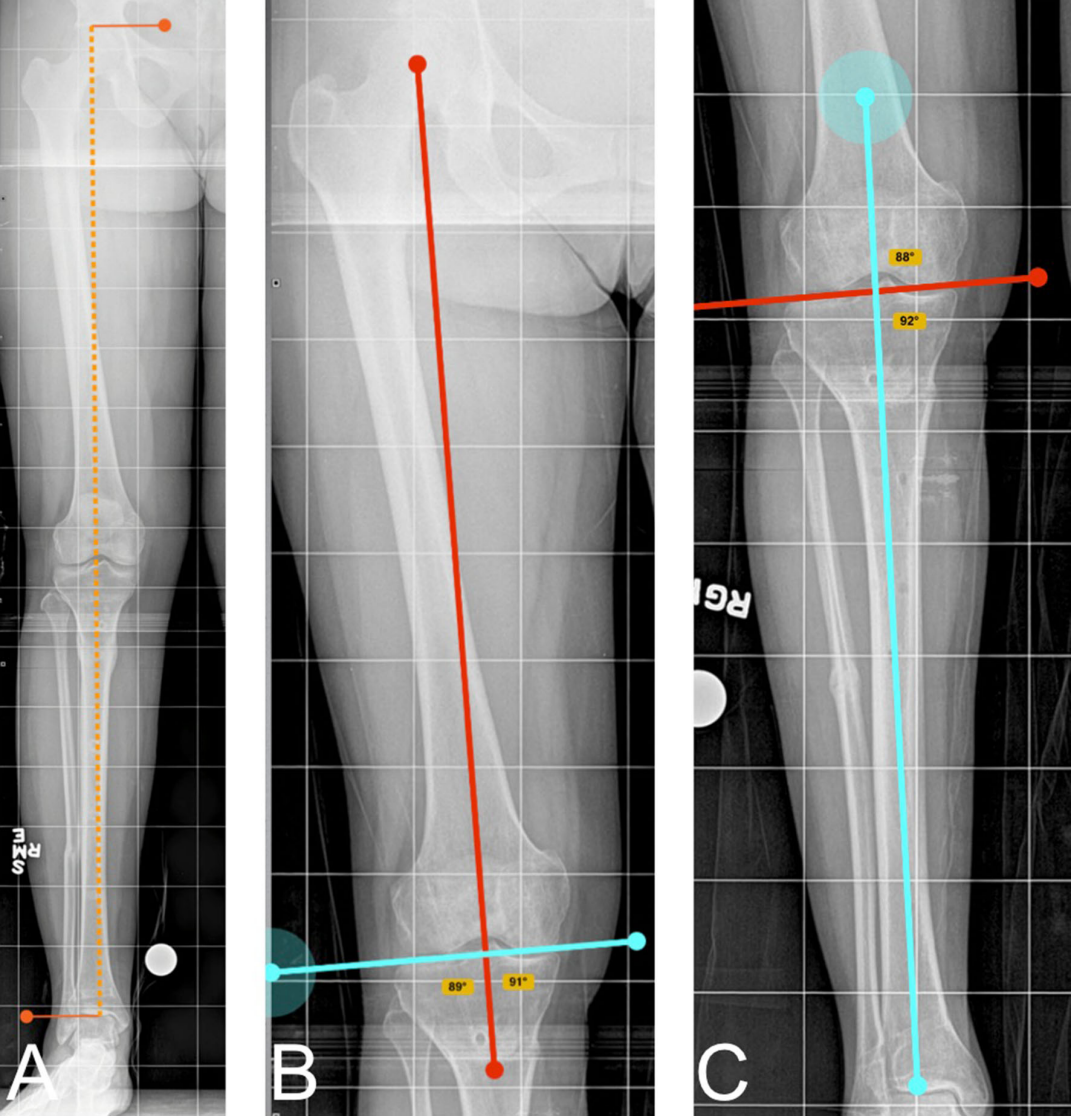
Radiographic measurements as demonstrated on Bone Ninja. a Total limb length. b Lateral distal femoral angle (LDFA). c Medial proximal tibial angle (MTPA)
